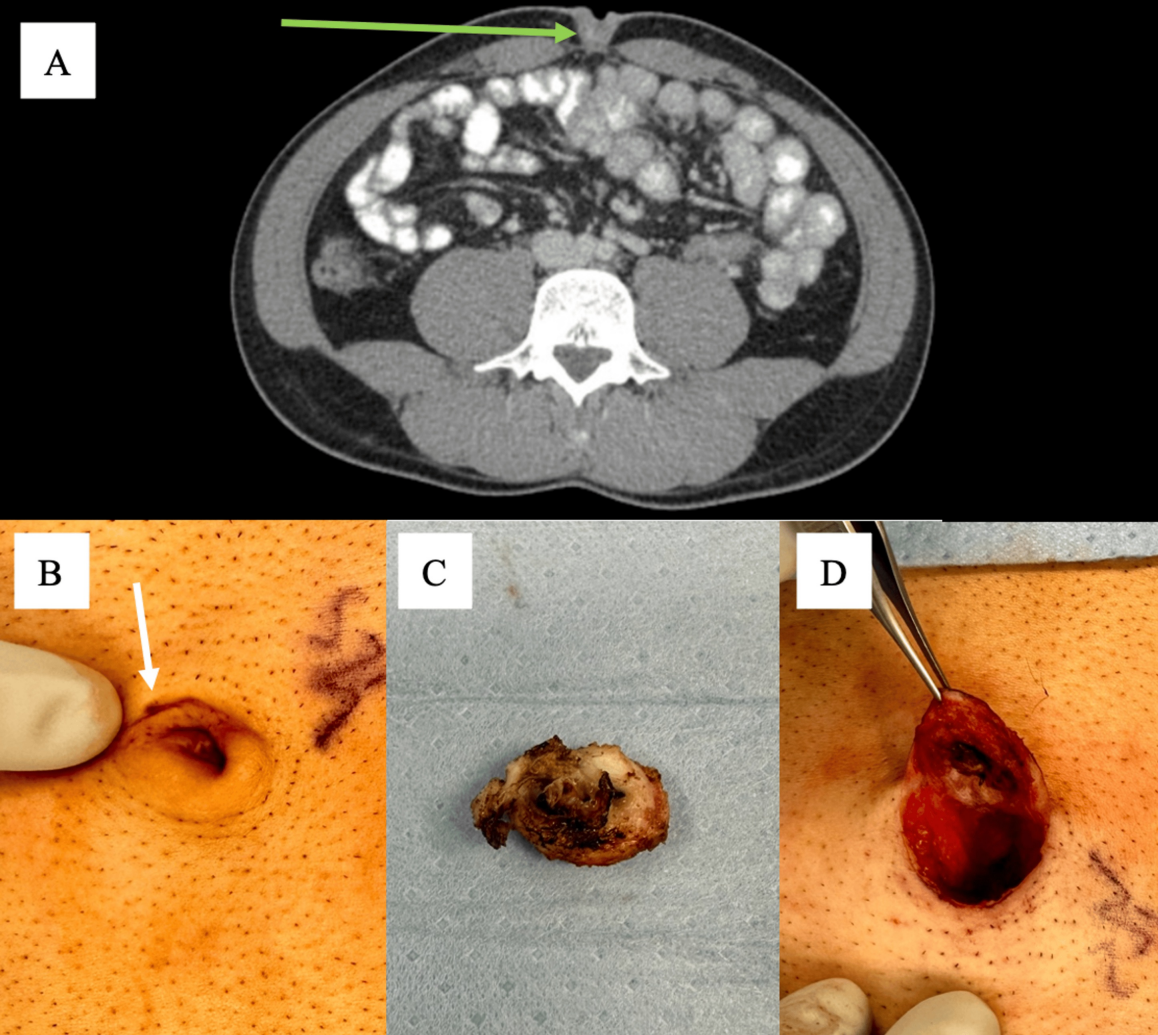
Umbilical pilonidal disease A: CT demonstrating umbilical inflammation (green arrow). B: Intraoperative photograph demonstrating pits (white arrow) surrounded by a large amount of shaved hair. C: Intraoperative photograph of resected umbilical pilonidal cyst, approximately 2.5cm in length. D: Intraoperative photograph of umbilicus following resection demonstrating resection cavity with overlying skin.
